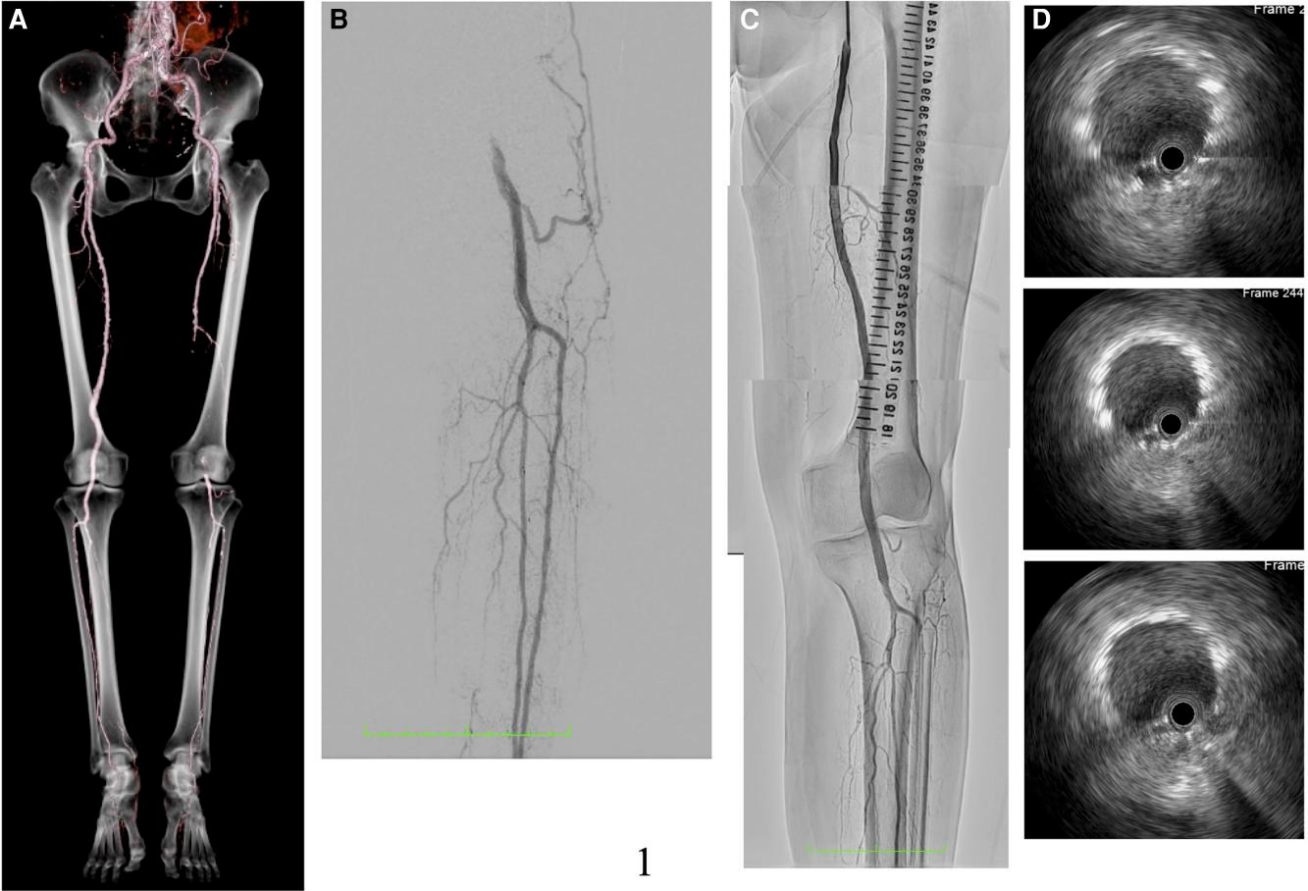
Computed tomography angiogram post-endovascular treatment (*A*). Control angiogram showed left popliteal artery occlusion from the left dorsalis pedis (*B*). The final angiogram revealed good blood flow from the superficial femoral artery down to the lesion below the knee (*C*). Intravascular ultrasound showed excellent expansion and apposition of the placed stents (*D*).
